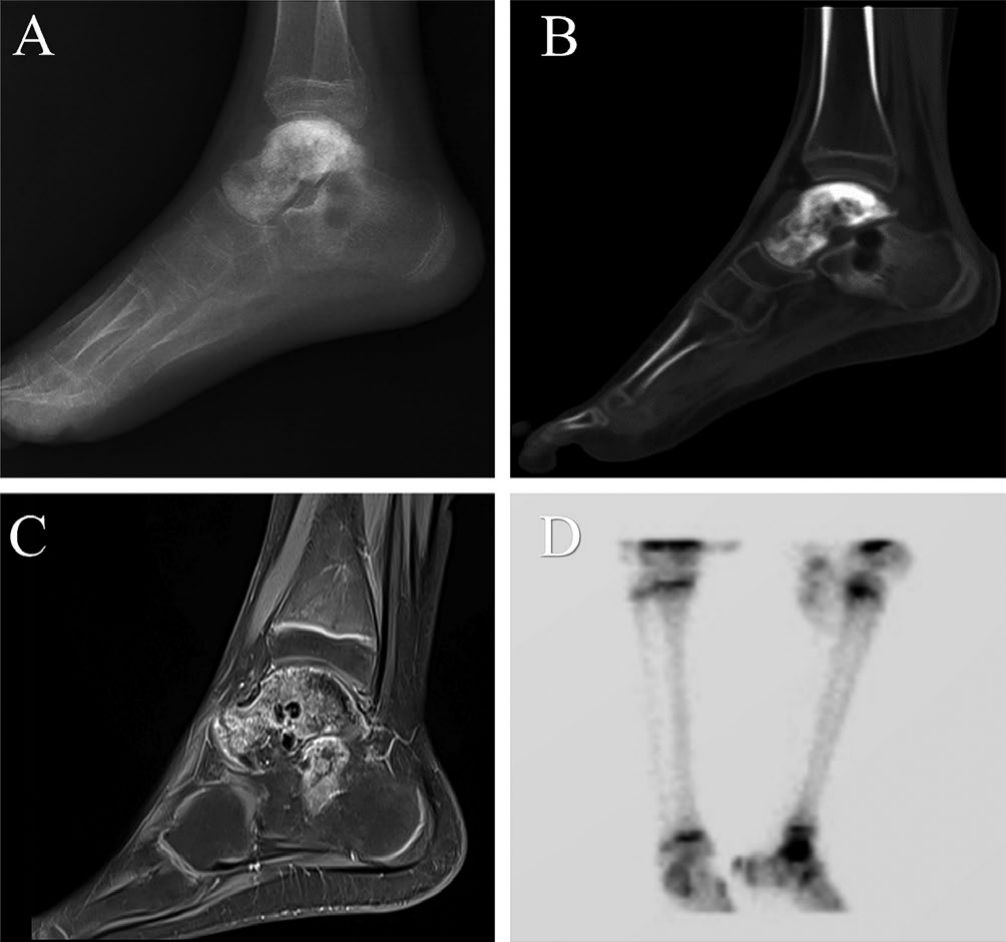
Preoperative radiograph (A), CT scan (B), MRI scan (C), and SPECT scan (D) of the patient with Ewing's sarcoma involving the talus, accompanied by suspected involvement of the calcaneus.
